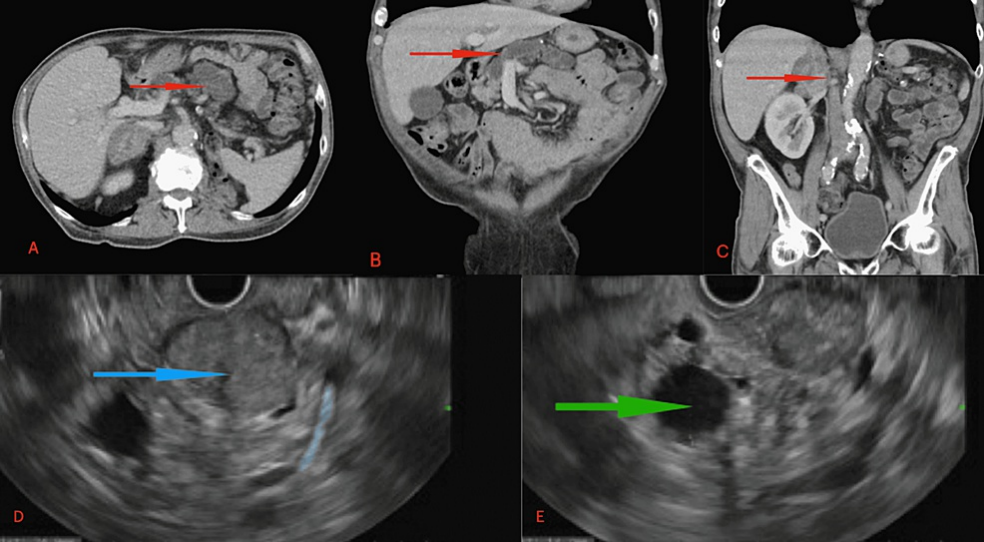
CT (panels A,B,C) and EUS (panels D,E) findings. Image A shows the mass in the pancreatic head (arrow with red head) with a dilated pancreatic duct seen in image B (arrow with red head). Adrenal metastasis is seen in image C (red arrow). On EUS, the mass appears well circumscribed in image D (blue arrow) with dilated pancreatic duct seen (green arrow) in the head of pancreas in image E EUS: endoscopic ultrasound (EUS)
